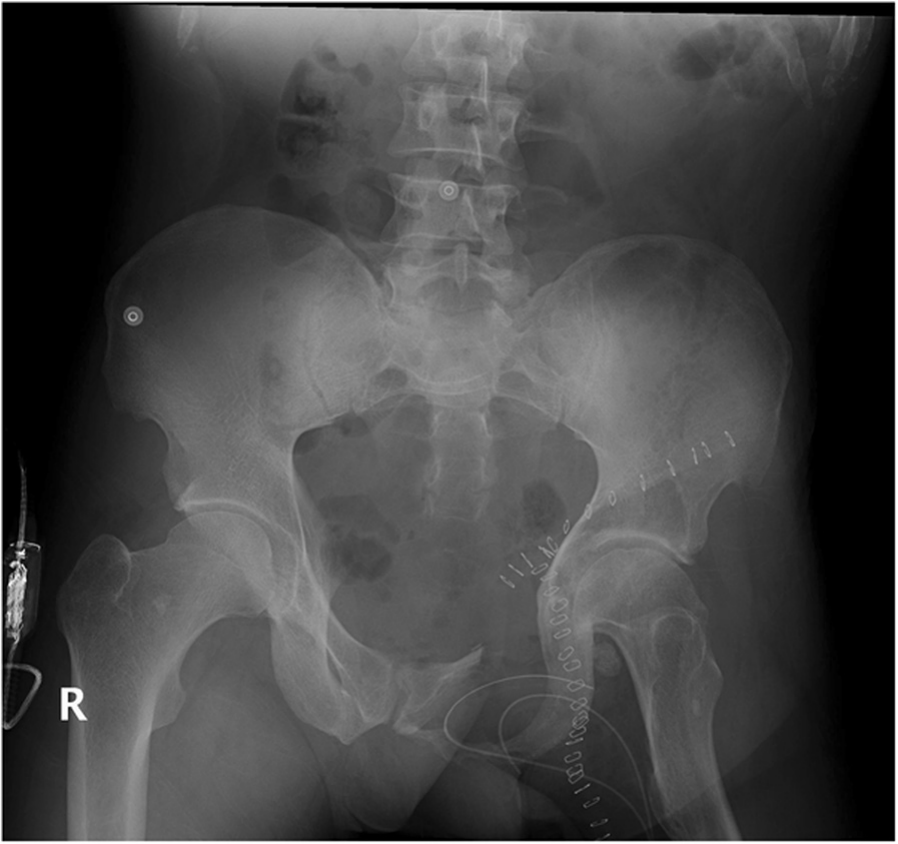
Post-resection radiograph depicts the bony defect and the ilio-inguinal incision.
